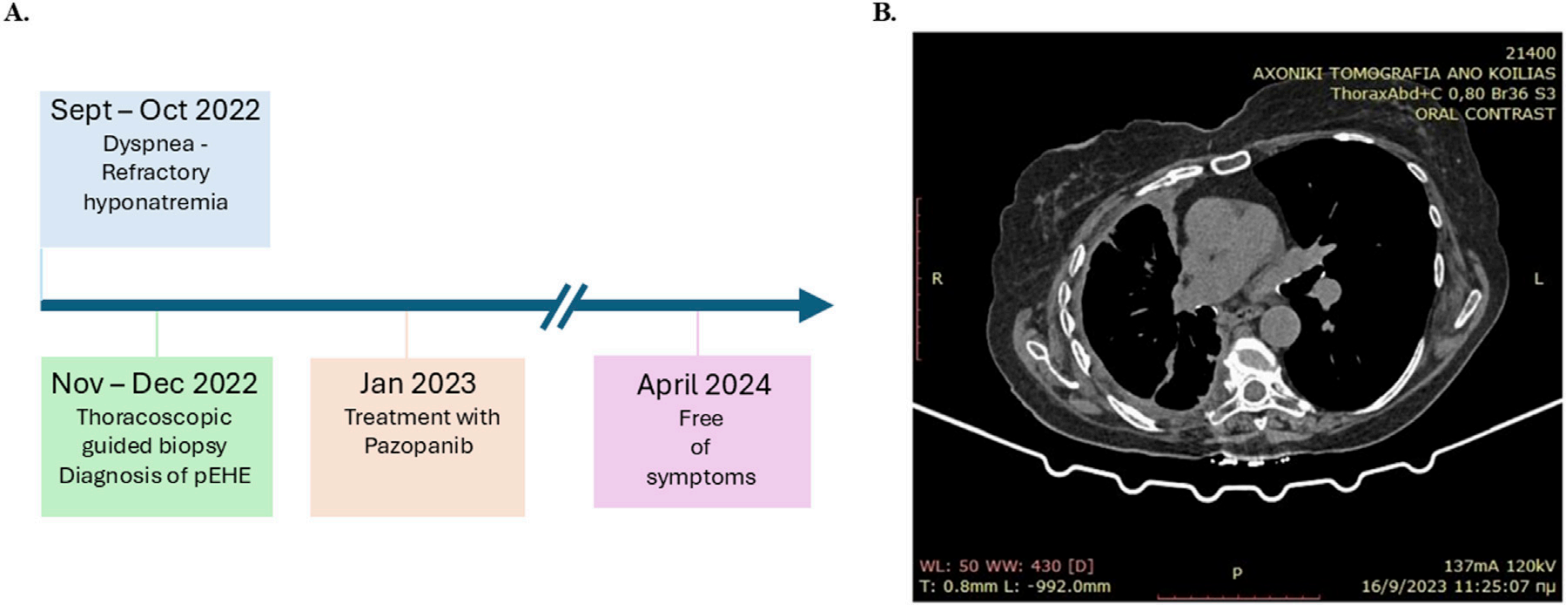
(A) Timeline showing the clinical course of the patient for pleural epithelioid hemangioendothelioma (pEHE) and post-diagnosis management. (B) Computed tomography (CT) scan obtained 9 months after commencement of first-line therapy.
